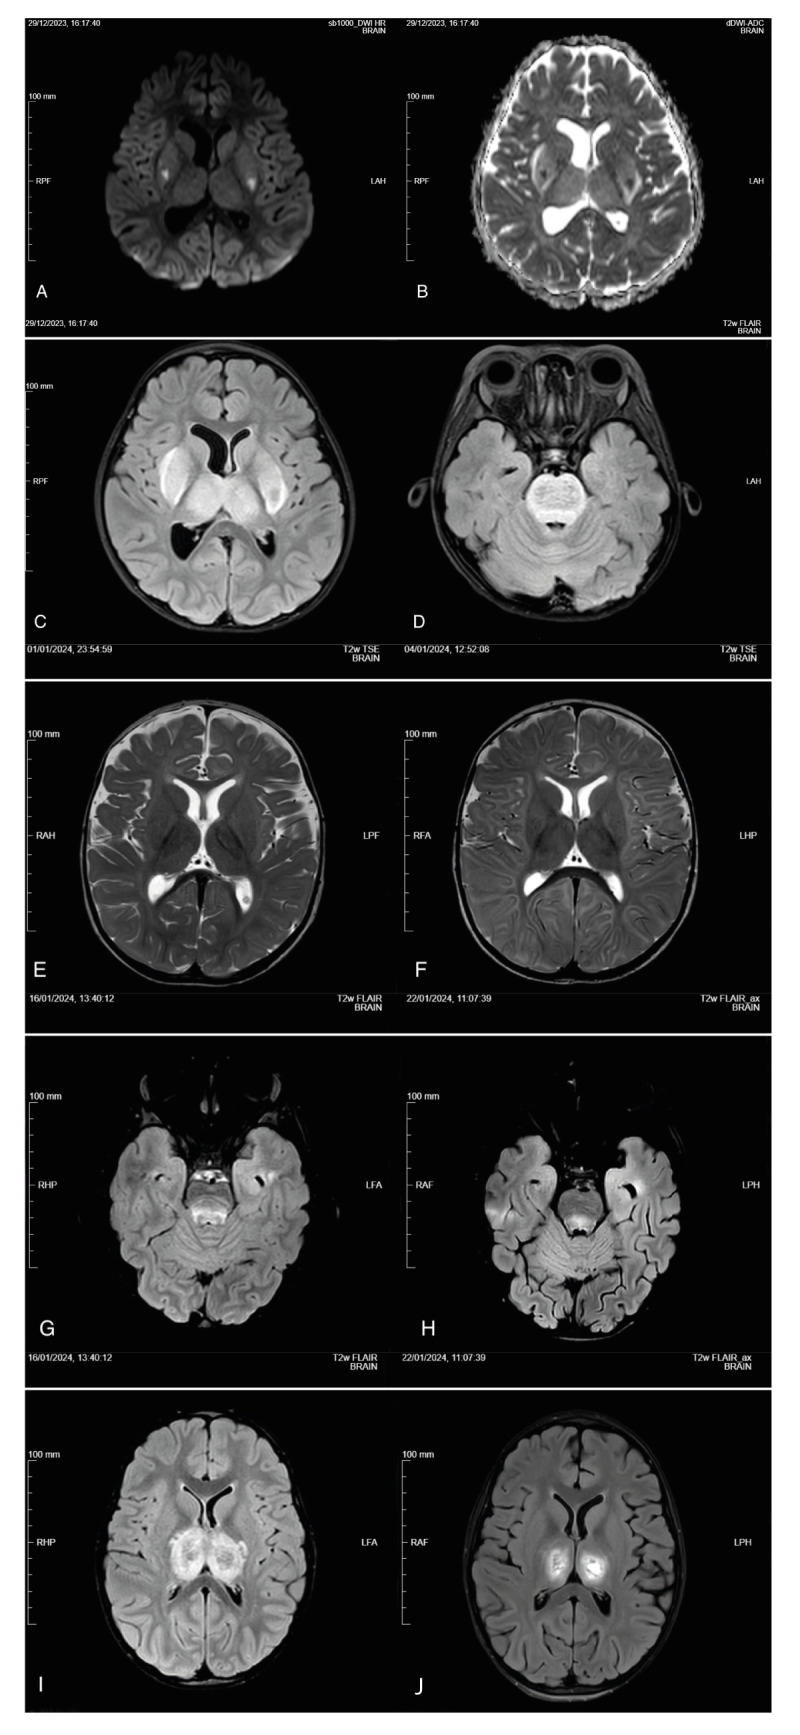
Brain magnetic resonance imaging in children with influenza A(H1N1)pdm09 acute necrotizing encephalopathy admitted to Meyer Children’s Hospital, Florence, Italy, December 2023–January 2024 (n = 3)
